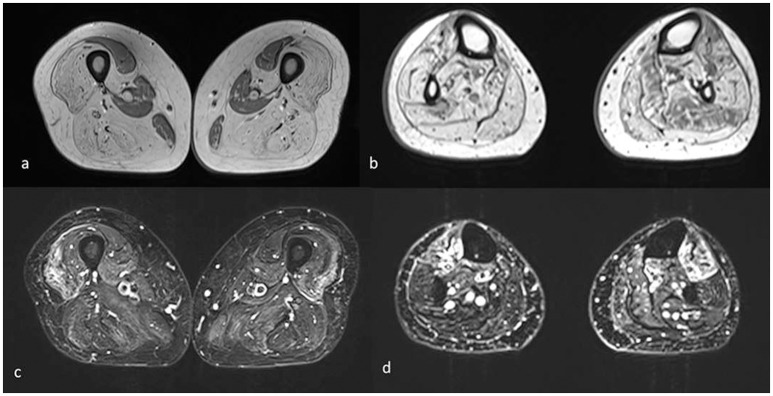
Muscle MRI axial T1 (a,b) and T2 STIR images (c,d) of the lower limbs shows mainly complete fatty substitution of both anterior and posterior thigh muscles, with selective sparing of the medial ones, specifically rectus, gracilis, sartorius, and adductor magnus (a). Diffuse adipose involvement also of the leg muscles, less pronounced at the level of the soleus, and tibialis anterior muscles (b). Inhomogeneous STIR hyperintensity was also associated, mainly in the quadriceps at the level of the thigh and in the tibialis anterior at the level of the leg (c,d).
